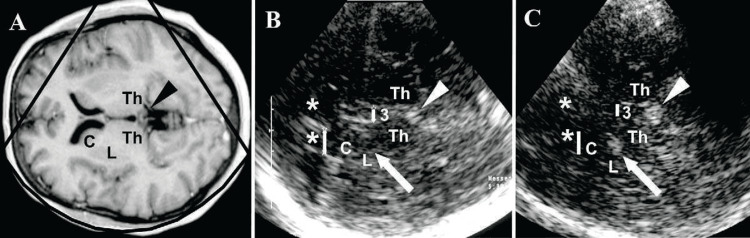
Transcranial sonography (TCS) of the brain at the level of the thalamus. (A) shows an MRI scan, while (B) and (C) show corresponding TCS images. Arrows in (B) and (C) correspond to lenticular nuclei. C: caudate nucleus; L: lenticular nucleus; Th: thalamus; 3: third ventricle (bar is indicating width); *lateral ventricle’s frontal horn (bar is indicating contralateral frontal horn’s width); pineal gland shown as a triangle.
